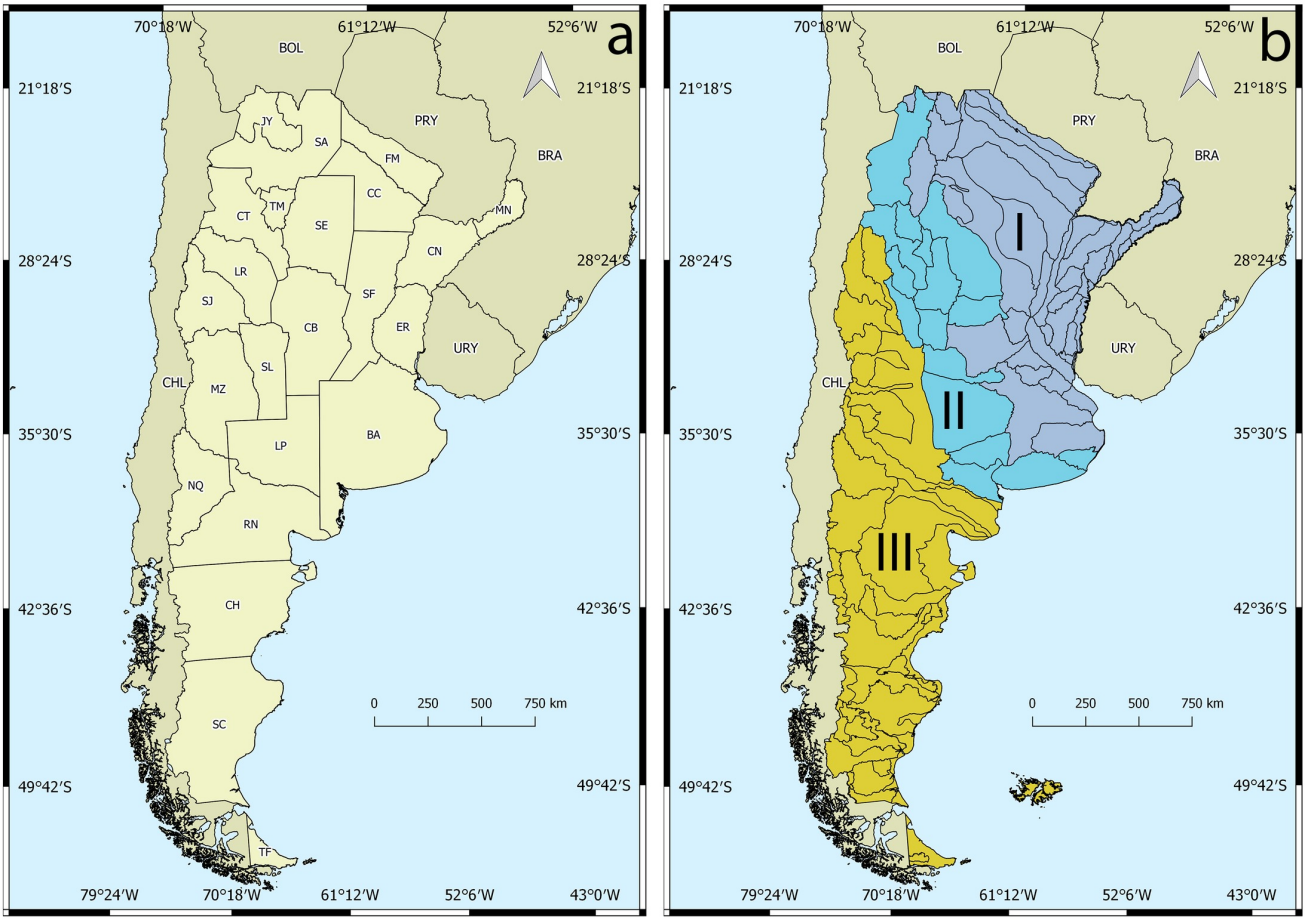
a) Political division of Argentina: Jujuy (JU), Salta (SA), Tucumán (TC), Formosa (FM), Chaco (CO), Misiones (MN), Corrientes (CN), Entre Ríos (ER), Catamarca (CT), San Juan (SJ), La Rioja (LR), San Luis (SL), Santiago del Estero (SE), Mendoza (MZ), Córdoba (CB), Santa Fe (SF), La Pampa (LP), Buenos Aires (BA), Neuquén (NQ), Río Negro (RN), Chubut (CH), Santa Cruz (SC), Tierra del Fuego, Antártida e Islas del Atlántico Sur (TF). b) Surface Drainage Basins of Argentina [40], [41] in South American Hydrographic regions [43]. I: The Plata basin, II: Border strip of the Brasilica (North) and the Chilean-Patagonian (South West) Subregion and III: Chilean-Patagonian Subregion of the Atlantic Versant.
